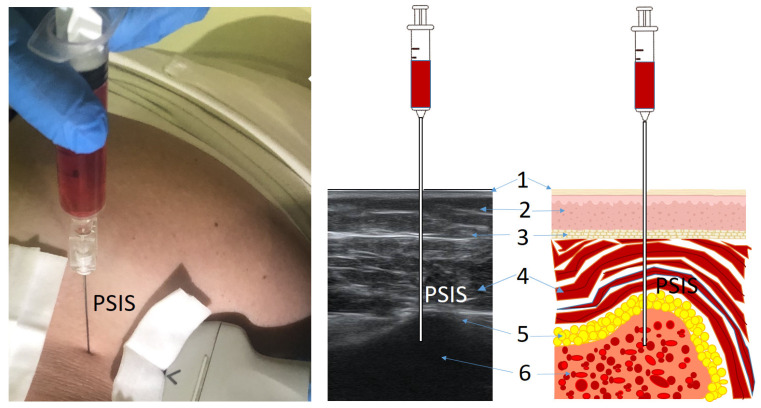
Ultrasound-guided intraosseous lidocaine trigger point injection technique. Notes: PSIS: posterior superior iliac spine; 1: epidermis/dermis; 2: subcutaneous tissue; 3: superior muscle fascia; 4: gluteus maximus; 5: periosteum; 6: spongey substance.
